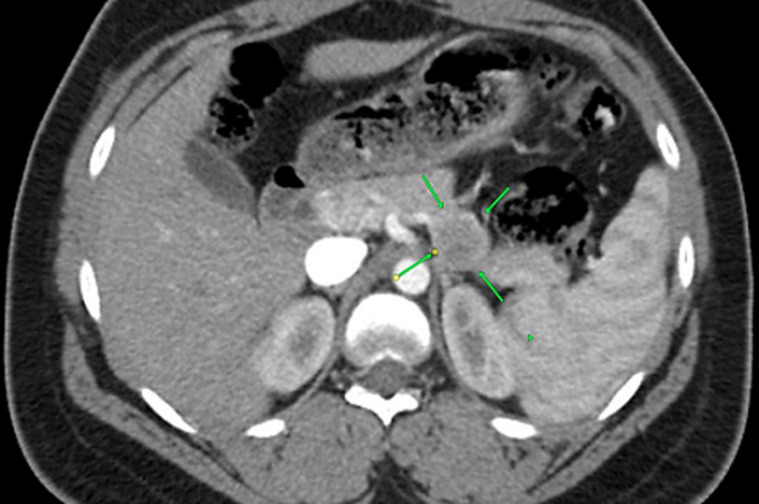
computed tomography, axial view of the tumour
